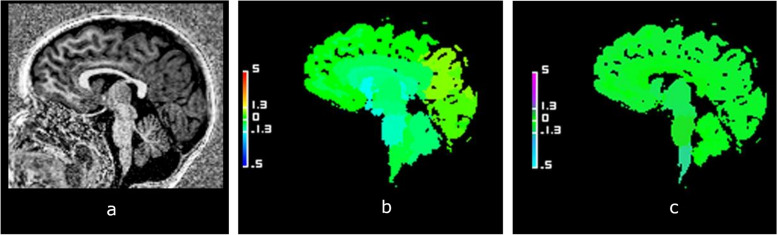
Example of a case of normal corpus callosum (CC). Sagittal view of the brain midline in a 3-year-old girl with neurodevelopmental delay. a High-contrast anatomical T1-weighted uniform image showing a normal CC. b Normal volumetric deviation map, with a CC appearing in green, Z-score = 0. c Normal T1 relaxometric deviation map, with CC appearing in green, Z-score = -0.52
